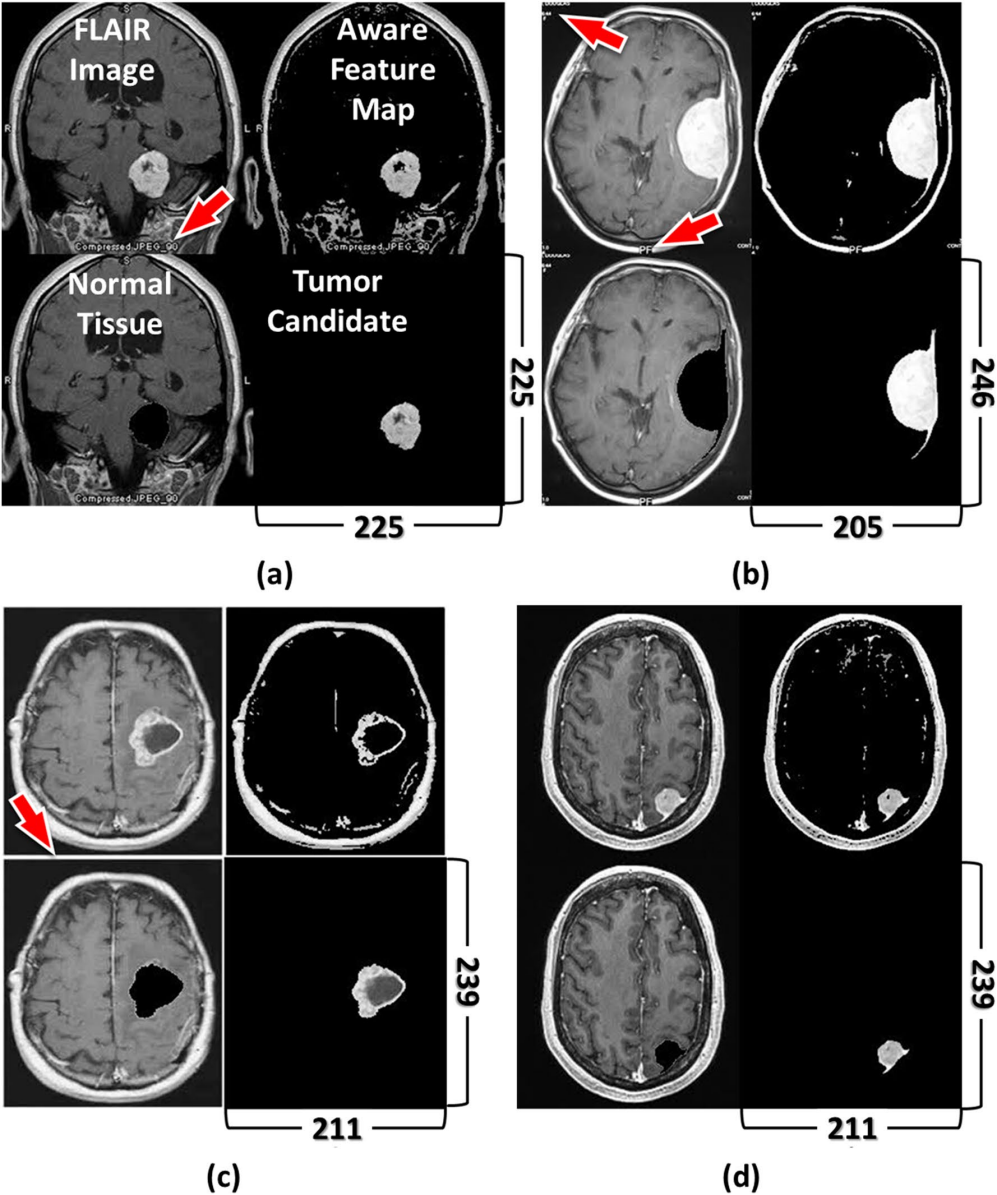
The performance of cases with interferences. As labeled in (a), the four sub-images of each panel indicate the FLAIR image (upper-left), the aware feature map (upper-right), normal tissue (lower-left), and the tumor candidate (lower-right). Image dimensions are next to the owning image in the lower right corner. The FLAIR images of (a) and (b) have notations added by radiologists (indicated by red arrows) and represent coronal and axial views, respectively. There is a white margin (indicated by a red arrow) in the original image of (c) and a relatively small tumor in (d). The CPU time for this small dataset ranges between 0.06 to 0.09 s. We acquired these images from an open-source dataset^33^.
